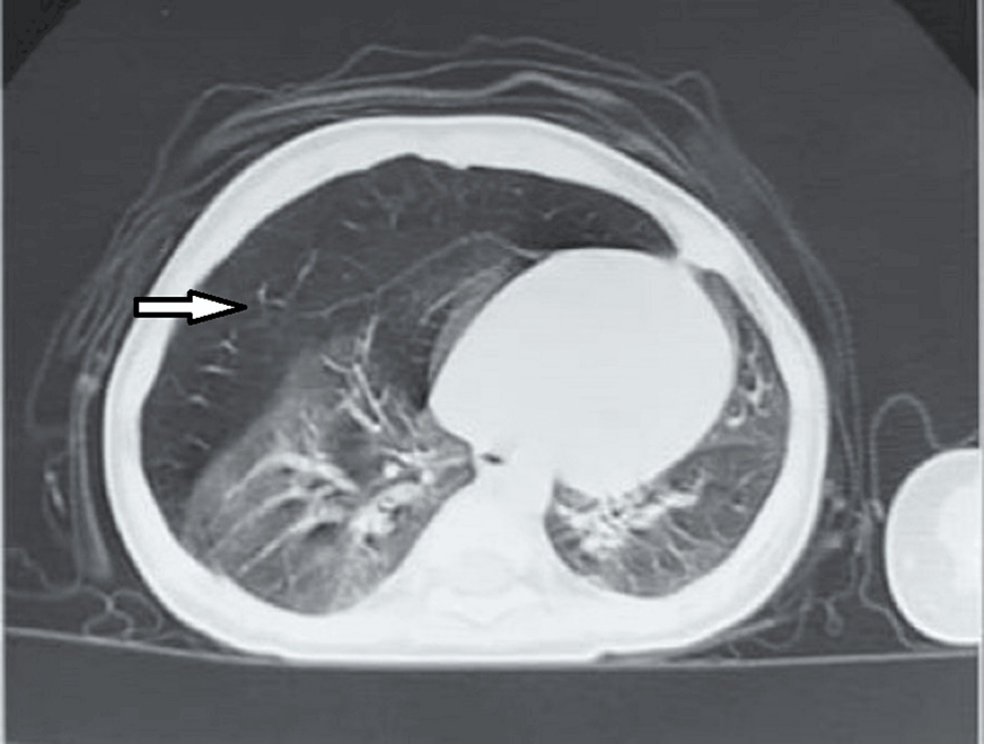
Chest CT showing right lobar emphysema
